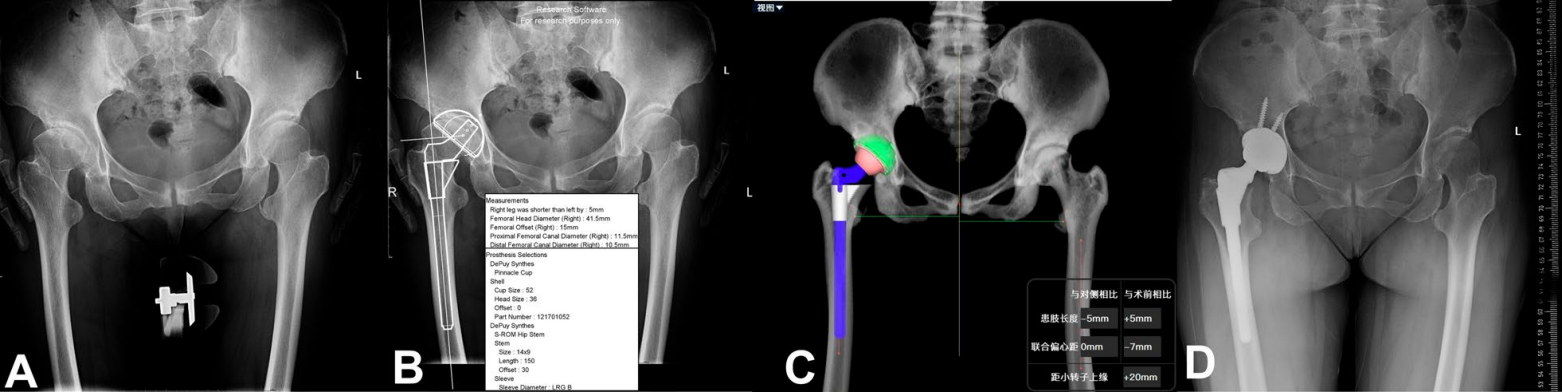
Case 1, woman, 54 years old, Crowe I DDH, left. A Standard preoperative anteroposterior X-ray of the pelvis. B Preoperative planning by the 2D template; planning results: Cup size was 50 mm. C Preoperative planning by AIHIP; planning results: Cup size was 50 mm. D Standard postoperative anteroposterior X-ray of the pelvis: Cup size was 50 mm
